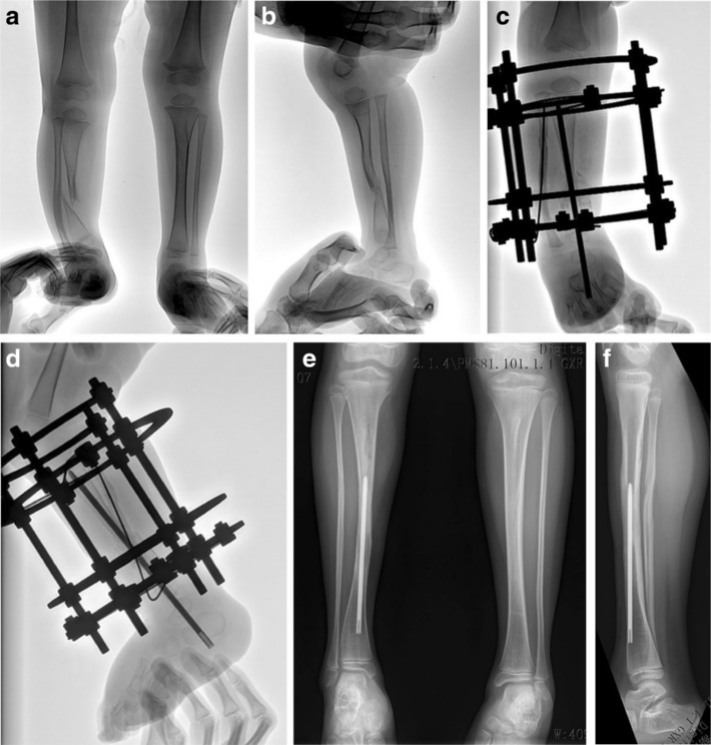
A typical case of 2.5 years boy with congenital pseudarthrosis of the tibia. Preoperative anteroposterior and lateral X-rays shows Crawford type IV CPT and intact fibula (a, b). Anteroposterior and lateral radiograph of the same patient taken at 2 months after combined surgey (c, d). X-ray of 5.4 years post operation shows solid union of the pseudarthrosis with good alignment of the tibia without ankle valgus, tibia angulation or LLD. The rod was in the tibia medullary cavity with growth without surgical pushing of the rod (e, f)
